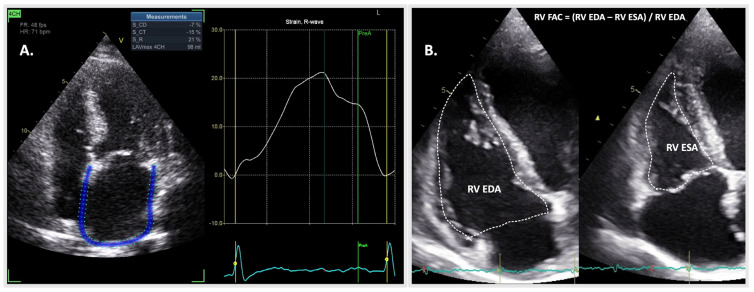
Methodology for right ventricular and left atrial assessment. (A). Measurement of left atrial strain using speckle-tracking echocardiography from the apical four-chamber view with the corresponding strain curve. (B). Assessment of right ventricular fractional area change (RV FAC), derived from RV end-diastolic area (EDA) and end-systolic area (ESA) tracings in apical four-chamber view. RV FAC was calculated as (RV EDA − RV ESA)/RV EDA. Both parameters were significant predictors of arrhythmia recurrence in the present cohort.
